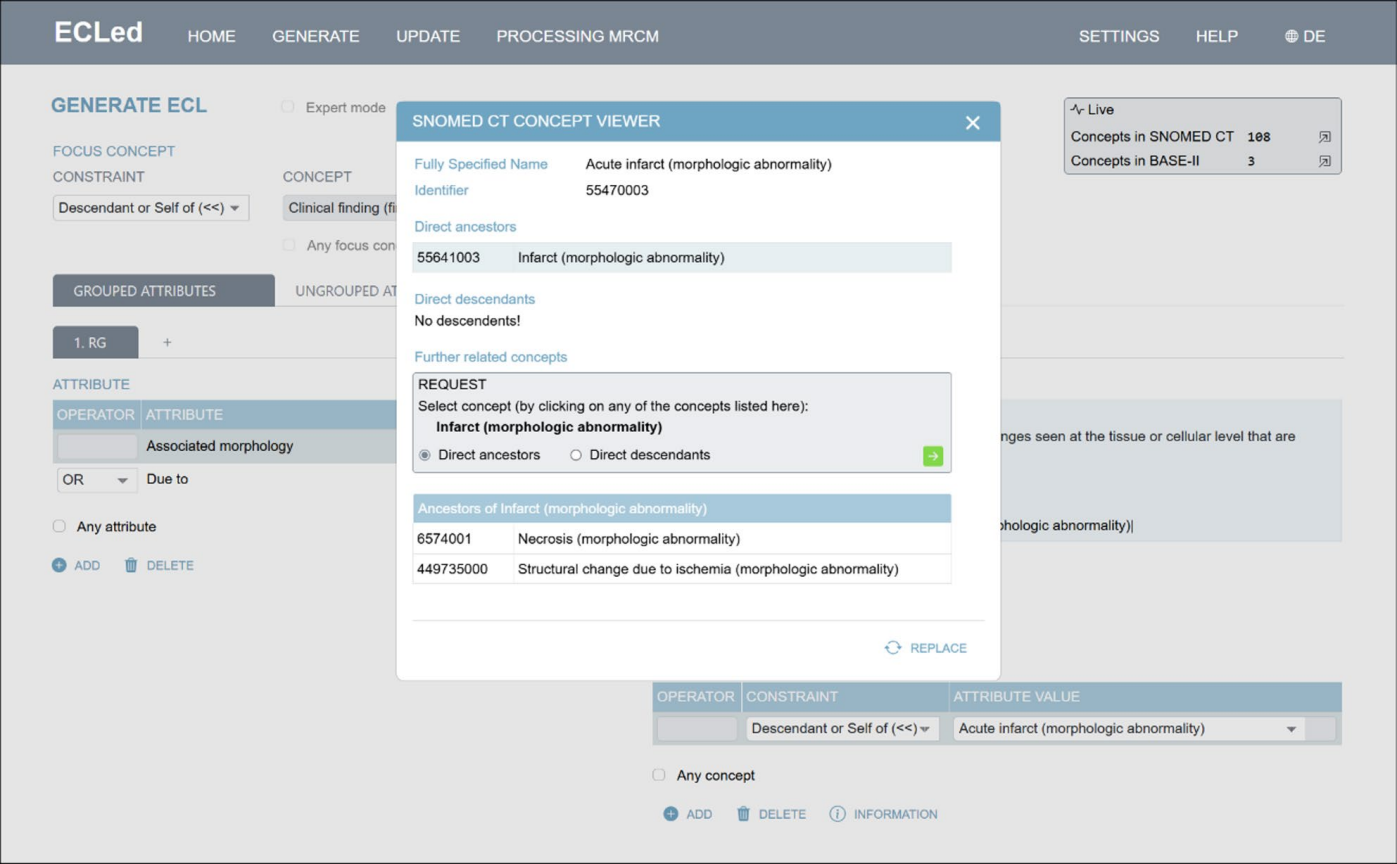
ECLed*’s SNOMED CT Concept Viewer showing selected concept with parent and child concepts in a modal window
